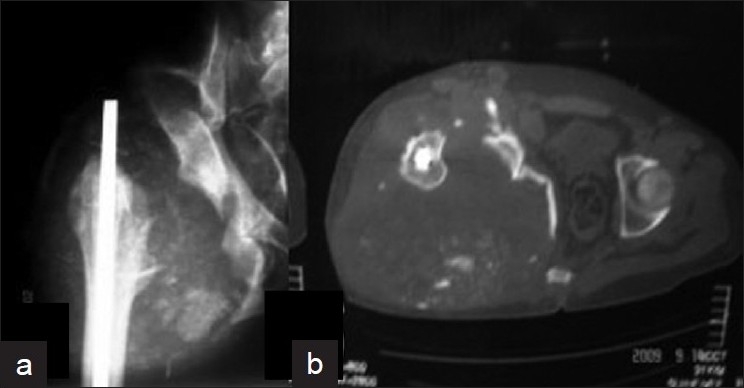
Anteroposterior radiographs (a) and computed tomography scan of hip (b) at time of presentation showing dramatic destruction of the right hip joint with fragmented bone debris in place
